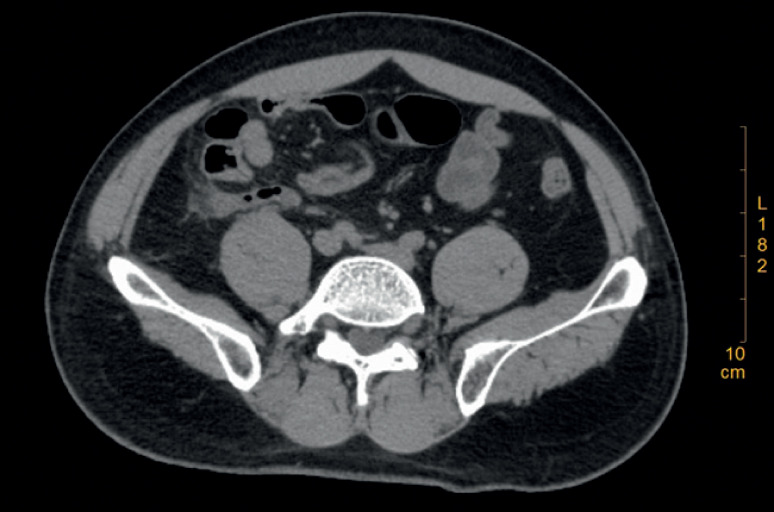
Postoperative computed tomography demonstrated fecaliths had been removed completely and the stent discharged.
